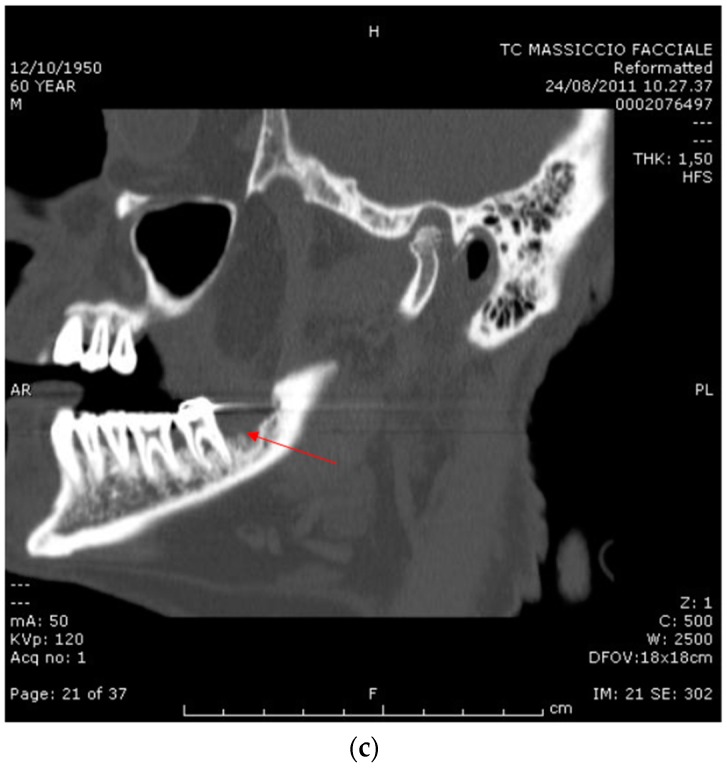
Case 2: CT scan images of the mandible post-extractive socket. (a) Coronal formatting; (b) Panorex; (c) Sagittal formatting that shows the bone sequestrum.
